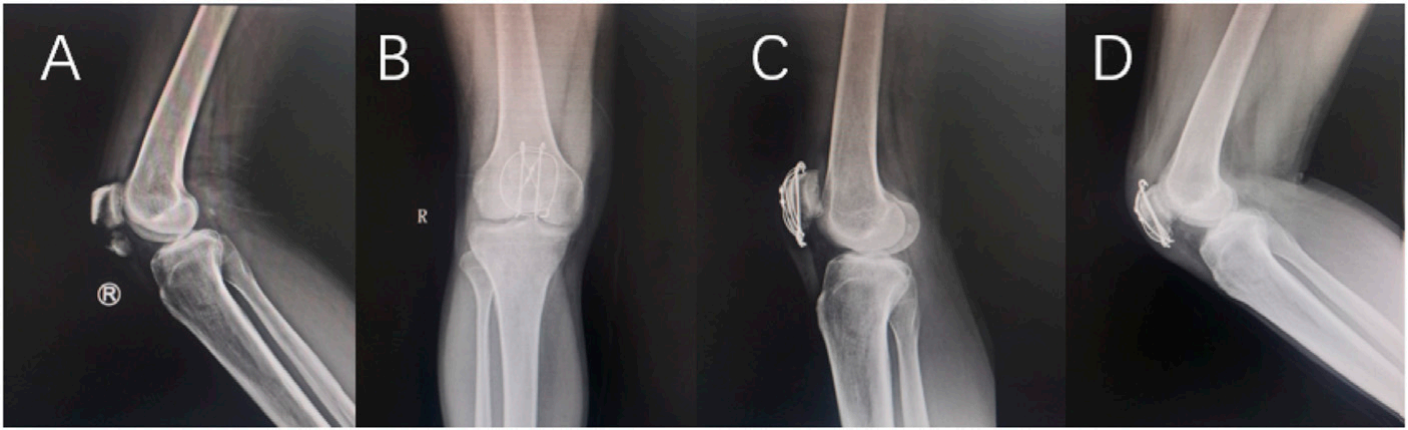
A 45-year-old man suffered a fracture of the inferior pole of the right patella due to a fall. Preoperative X-ray (A), postoperative knee joint X-ray (B,C). The X-ray film (D) was reexamined 9 months after operation.
